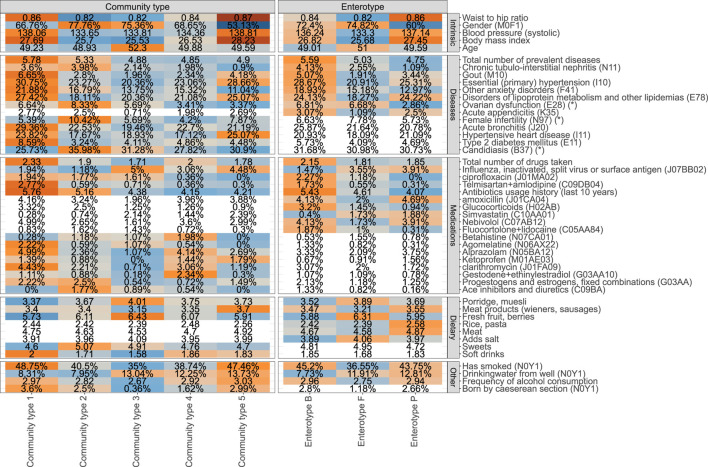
Phenotype associations with the enterotype (ET) model and community type (CT) model (unadjusted analysis). Coloured cells represent factors associated with CT and ET models respectively (FDR ≤0.1), and white cells indicate no statistically significant association (FDR > 0.1). Mean values or proportions (indicated by %) per cluster are shown. Blue colors indicate lower mean values or proportions for the cluster and orange color indicates higher values. Asterix (*) in the names of the factors indicate that a subpopulation consisting of women was used for calculating the displayed value.
